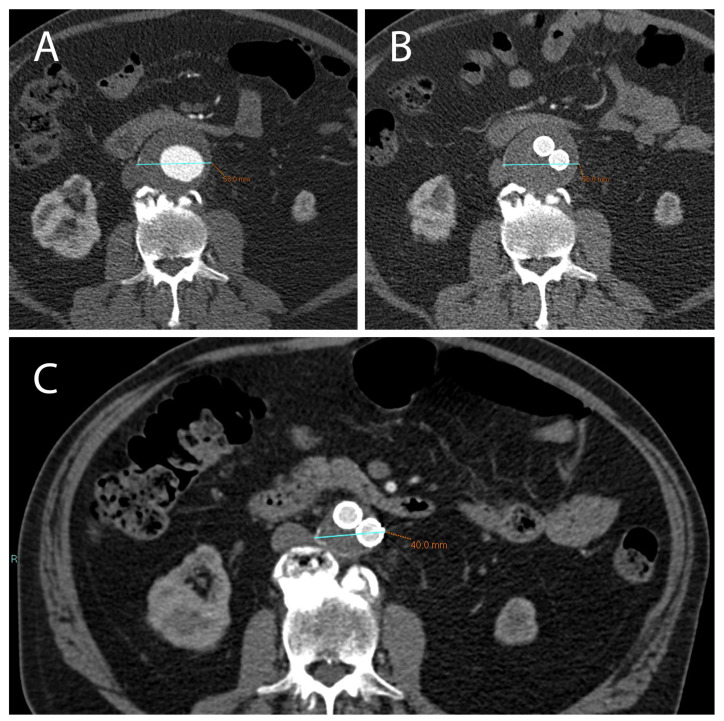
(A) CTA axial images show an AAA with a diameter >5.5 cm (in this case 5.8 cm) which can be treated with EVAR. (B) After 1 month following EVAR implantation, CTA was used to assess endoleak occurrence; of note, there was no significant reduction in AAA diameter in this short time-range. (C) After 12 months following EVAR intervention, a correct aneurysmatic sac exclusion without endoleak led to a significant reduction in AAA diameter (in this case the aneurysm diameter went from 5.8 cm to 4 cm).
